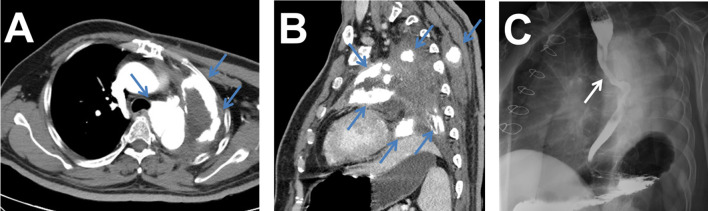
(A, B) Six months after the operation, contrast-enhanced CT scan showing enlargement of the recurrence and metastases (blue arrows). (C) Esophageal barium radiography showing that the tumor at the left hilum had compressed the adjacent esophagus (white arrow).
